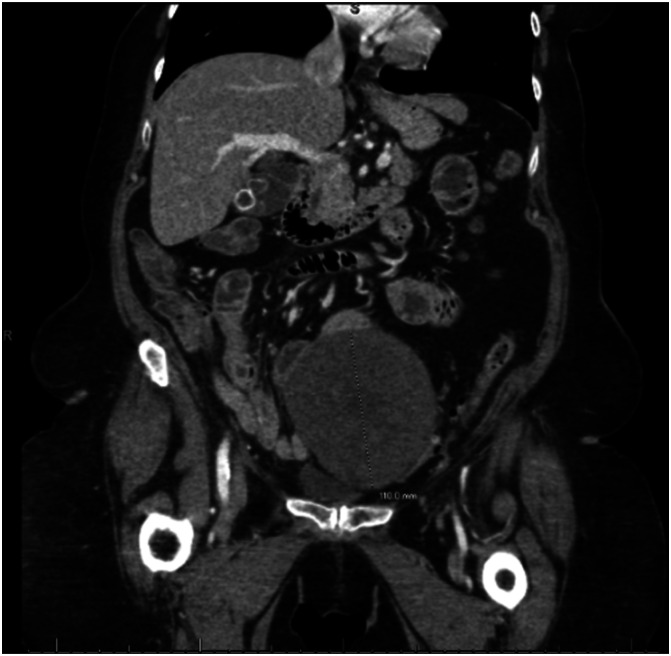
Computed tomography scan demonstrating the initial 14.6-cm pelvic mass in 2013.
